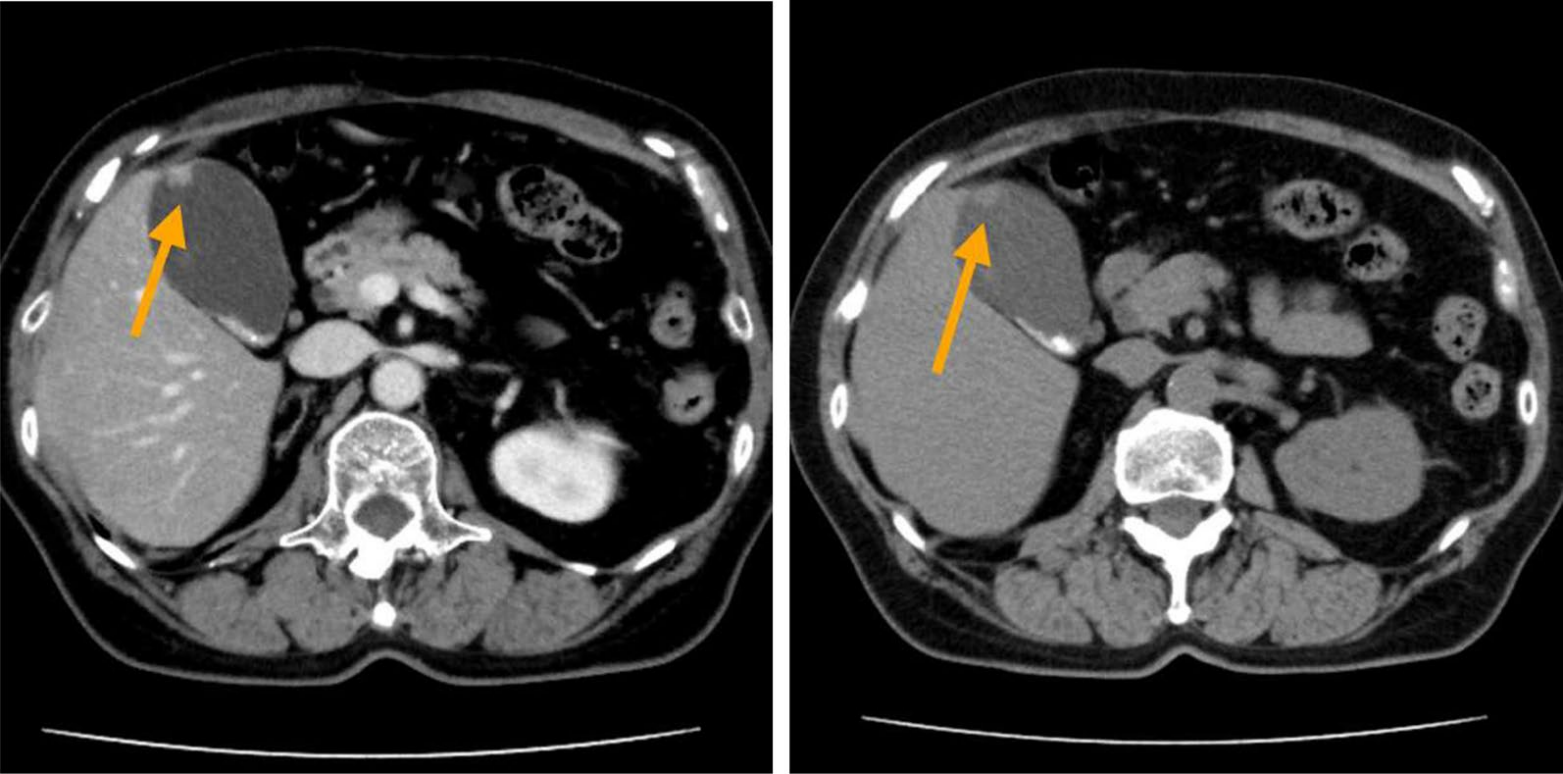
Abdominal computed tomography showed slightly enhanced tumor in the fundus of gallbladder (arrow)
